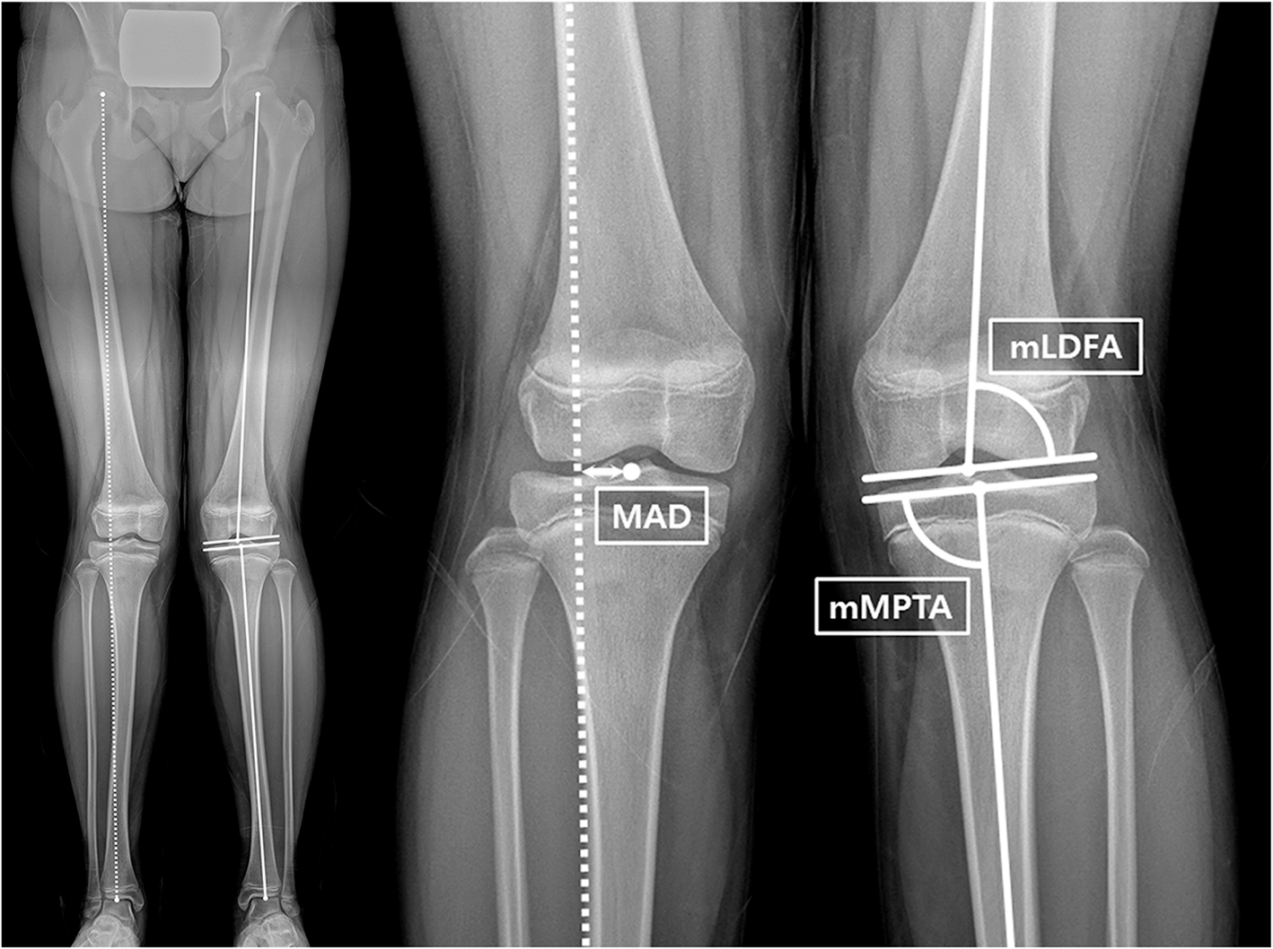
Radiographic measurements of mechanical axis deviation (MAD), mechanical lateral distal femoral angle (mLDFA) and mechanical medial proximal tibial angle (mMPTA).
